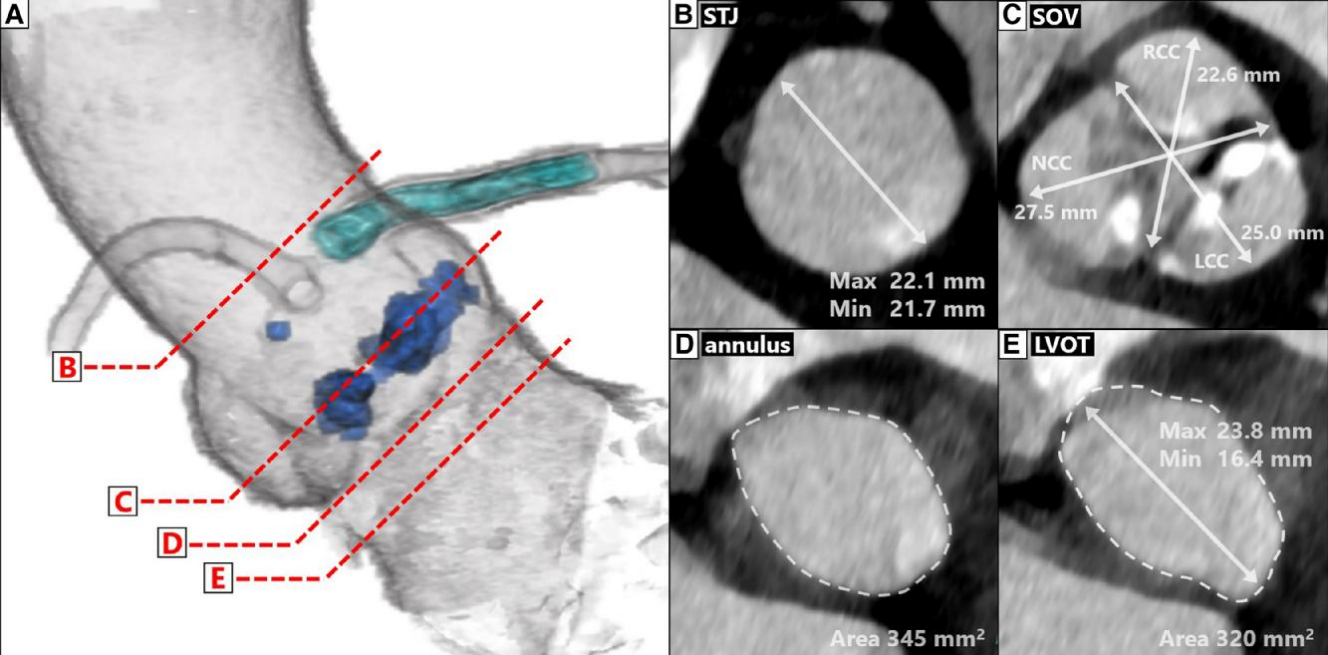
The aortic valve complex as seen on the preoperative computed tomography. In the perpendicular view (*A*), sections B–E showed the sinotubular junction (*B*), the sinus of Valsalva (*C*), the aortic annulus (*D*), and the left ventricular outflow tract (*E*), respectively. CT, computed tomography; STJ, sinotubular junction; SOV, sinus of Valsalva; LVOT, left ventricular outflow tract; RCC, right coronary cusp; LCC, left coronary cusp; NCC, non-coronary cusp.
